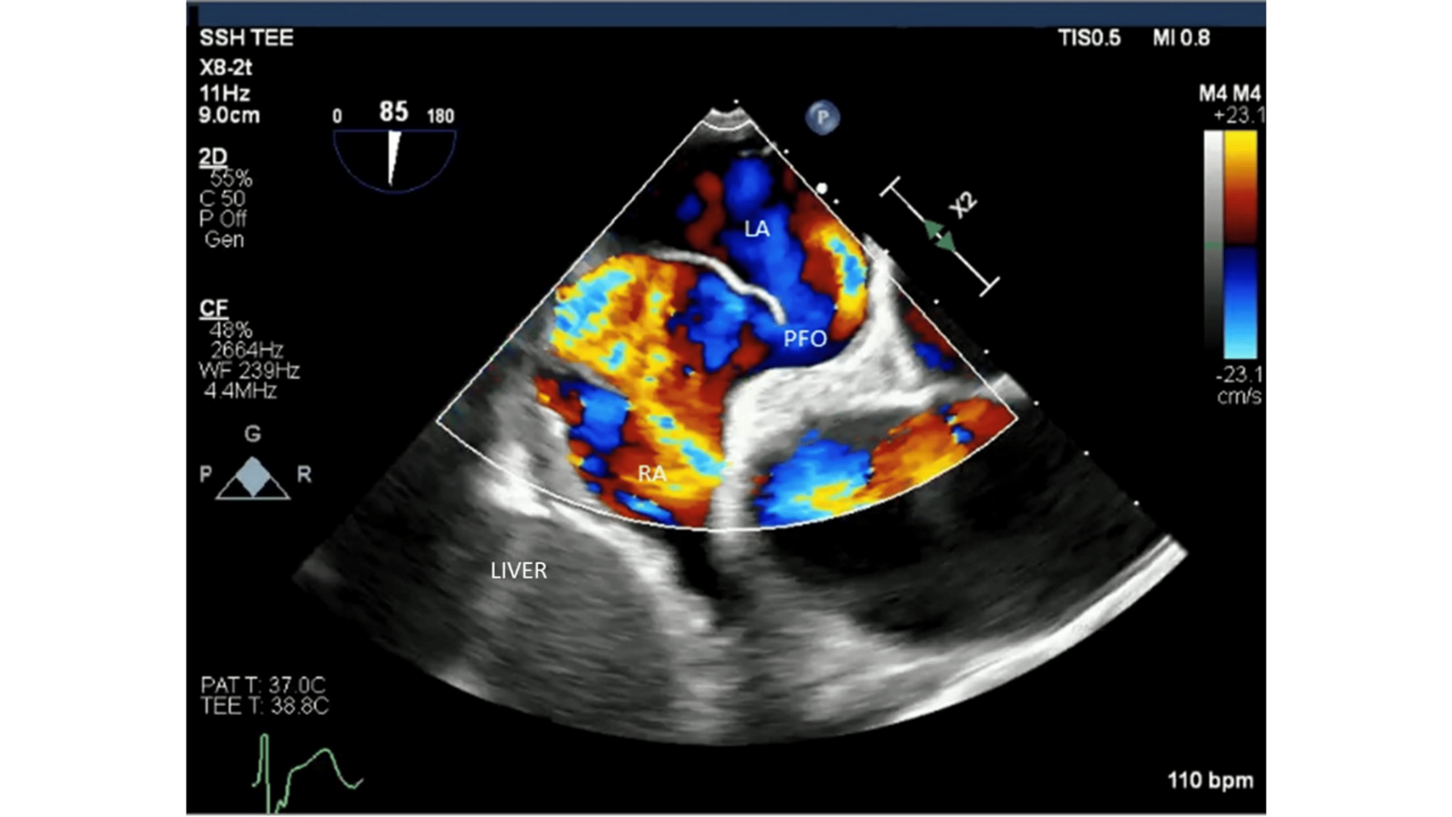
TEE bicaval view with color Doppler depicting PFO. The photo was captured during a trans-esophageal echocardiogram where a scope was inserted into the patient's mouth and down the esophagus to acquire views of the heart's chambers. The bicaval view of this echocardiogram allows visualization of the left atrium, right atrium, inter-atrial septum, superior vena cava, and inferior vena cava. The area within the right atrium with colors yellow, red, and blue indicates an area of high turbulence as blood moves between the high-pressure left atrium to the low-pressure right atrium via the PFO conduit in the color Doppler mode of echocardiogram capture. TEE: Transesophageal Echocardiogram; LA: Left Atrium; PFO: Patent Foramen Ovale; RA: Right Atrium
